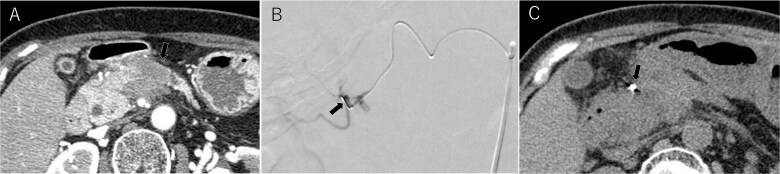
A 76-year-old female with pancreatic cancer. (A) Contrast-enhanced CT shows pancreatic cancer at the pancreatic body (arrow). (B) A branch of the anterior superior pancreaticoduodenal artery near the tumor was selected using a microcatheter, and a straight coil was placed as a fiducial marker (arrow). (C) Postprocedural CT shows an implanted fiducial marker beside the pancreatic cancer without migration (arrow).
